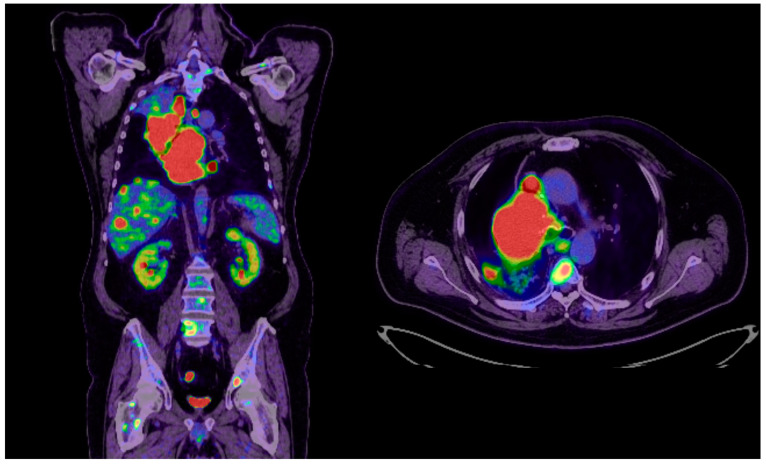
PET-CT (December 2023): A bulky tumor mass in the right mediastinal–pulmonary area that includes the right main bronchus, with maximum dimensions of 13.4/10.2/8.5. Multiple bilateral pulmonary micronodules. Liver with greatly increased dimensions, almost completely occupied by multiple nodular and macronodular lesions, the largest with dimensions of 68/45 mm, compatible with secondary determinations. Two lesions in the pancreas, the largest of 25/20 mm. Bulky adenopathies in the celio-mesenteric and retrocrural areas, the largest inferior to the perigastric cardia and 25/20 mm in dimension. The skeletal system: multiple metabolically active lesions. Disseminated metastatic oncologic disease with secondary determination in the lungs, supra- and subdiaphragmatic ganglia, liver, pancreas, muscles and bones.
